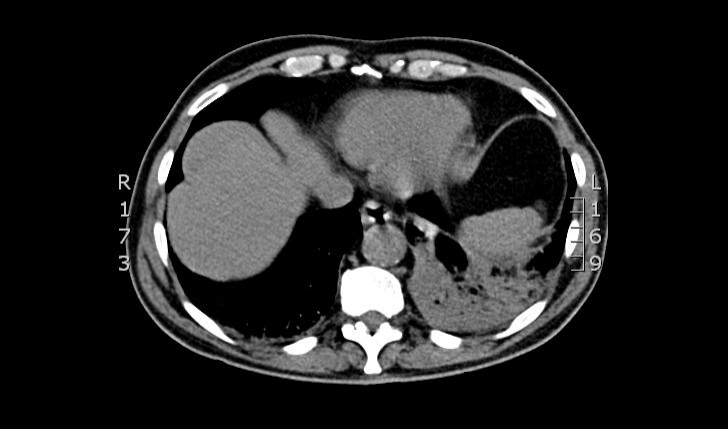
CT thorax demonstrating extensive lower lobe consolidation and collapse noted in the left lung with extensive hilar lymphadenopathy.
